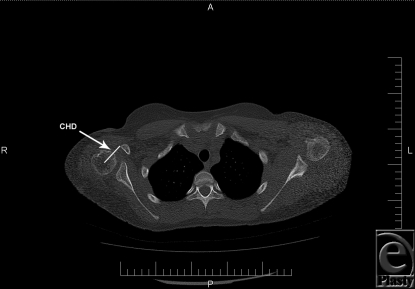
Postoperative computed tomography of the same patient showing coracohumeral distance, labeled “CHD.” Scale is shown at bottom. Space between each hache mark is 10 mm. CHD = 40 mm.
